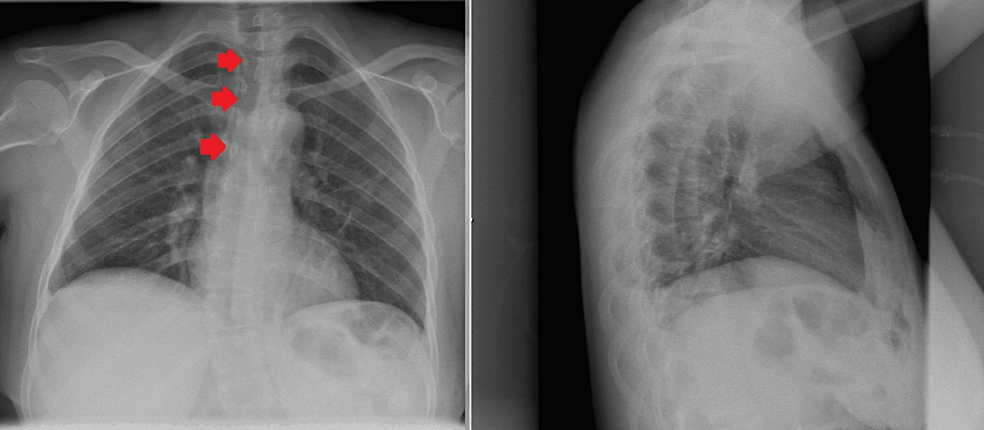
Chest X-ray Showing Pneumomediastinum Red arrows point to air in the mediastinum.
